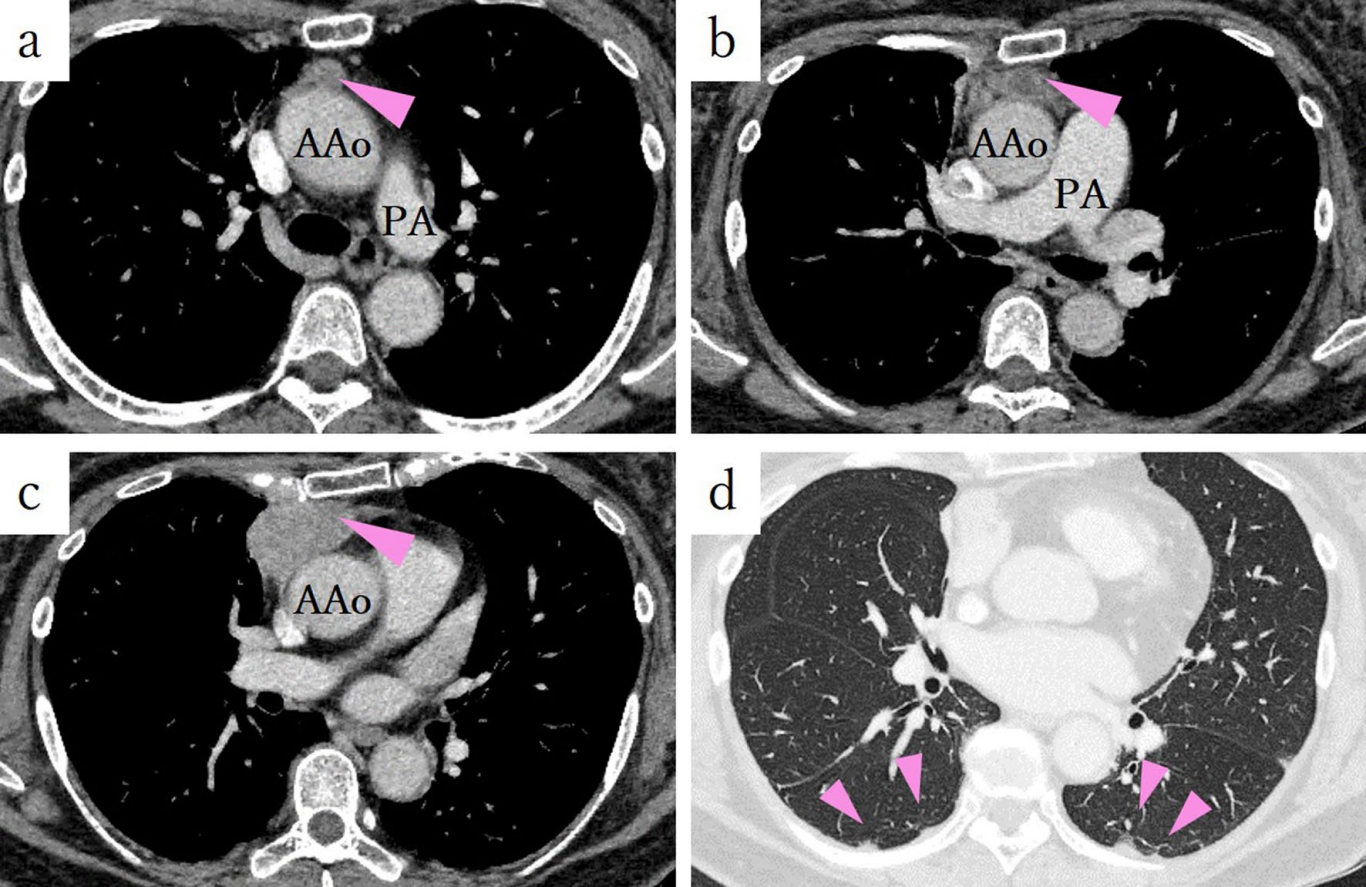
Preoperative CT shows tumors in the anterior mediastinum, measuring 15 × 14 × 10, 25 × 12 × 12, and 43 × 33 × 24 mm from the head (a, b, c). The two lower areas appeared to be continuous. b, c These findings suggested multicentric thymoma or intra-thymic metastasis. Furthermore, based on the tumor location, pericardial invasion, pleural dissemination (d), and a possible left adrenal adenoma were suspected. *AAo* ascending aorta, *PA* pulmonary artery, *CT* computed tomography
